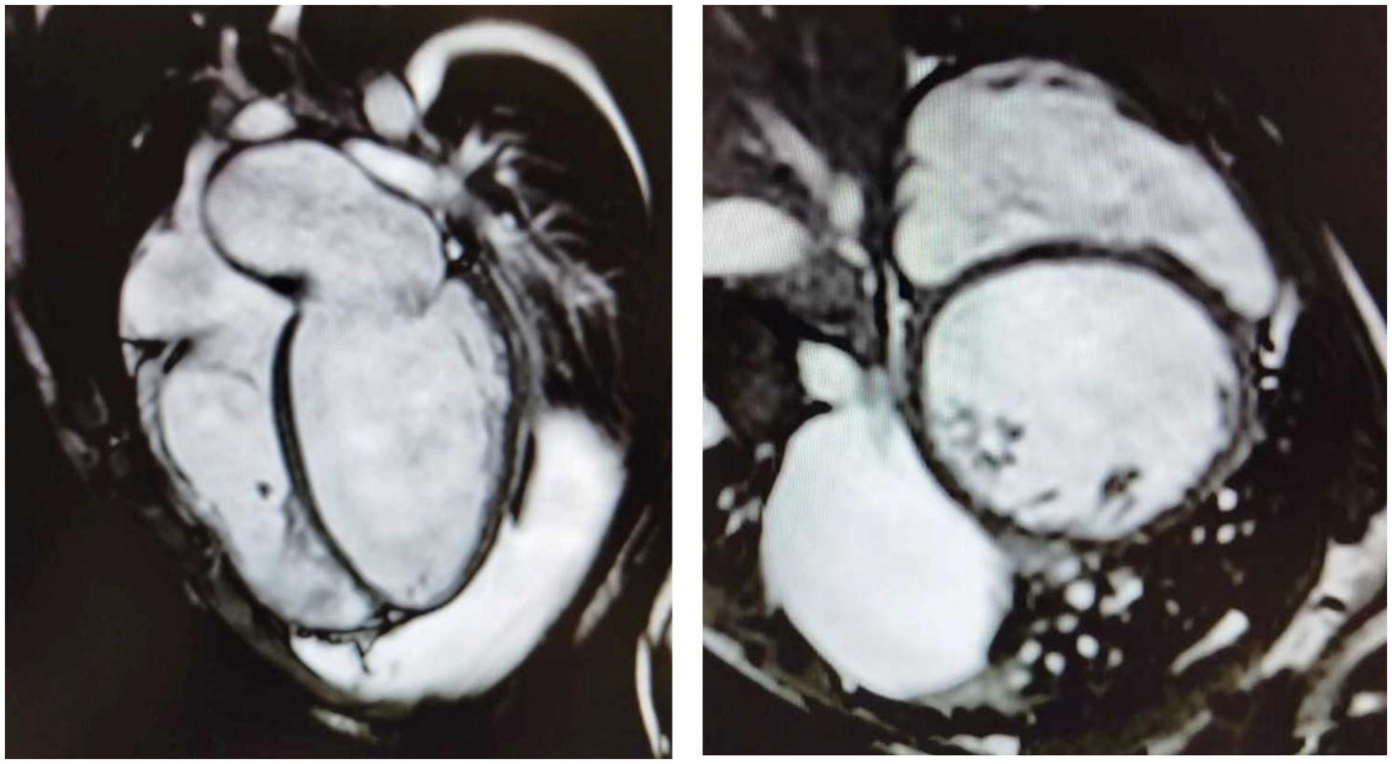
Four-chamber (left) and short-axis (right) cine images from cardiac magnetic resonance imaging demonstrating severe bi-ventricular dilatation, accompanied by myocardial wall thinning and fibrosis.
